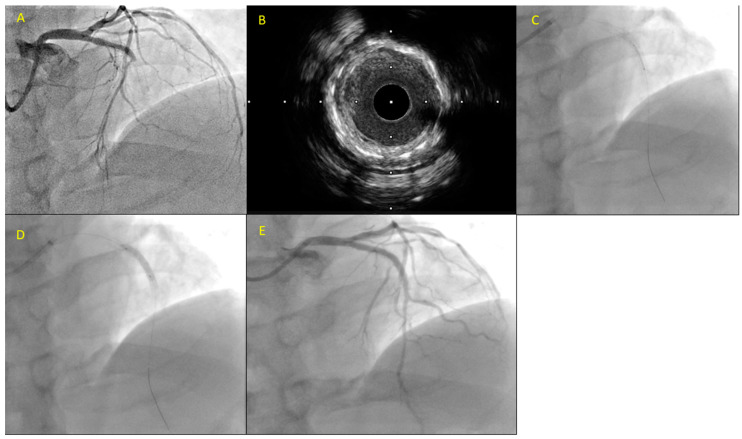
A fifty-three-year-old patient presented with substernal chest pain and ST-segment elevation in leads V2 through V6. He had a history significant for anterior STEMI with a DES placement in the LAD 3 years prior. The patient was led to the catheterization laboratory where he was diagnosed with in-stent thrombosis (A). After predilations with a 2.5 mm non-compliant balloon, intravascular ultrasound (IVUS) was performed showing stent underexpansion and neoatherosclerosis. (B) A new DES, a 3.0 mm × 12 mm DES, was delivered inside the previously implanted stent (C) followed by postdilations with a 3.5 mm non-compliant balloon (D) with a good final result (E).
